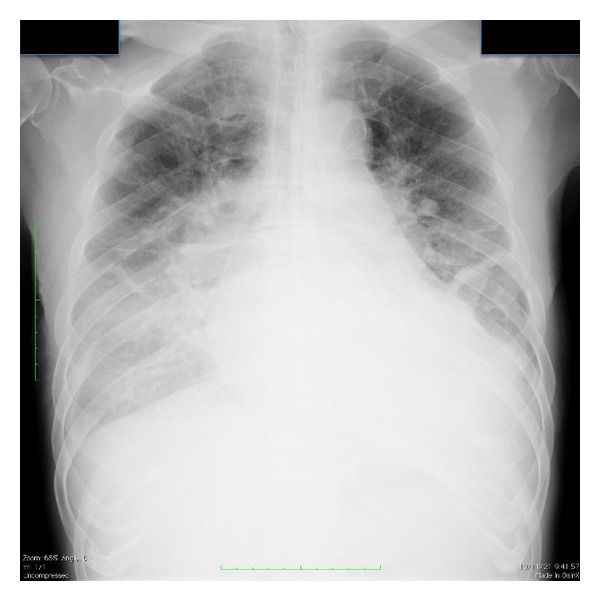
Chest X-ray at admission: significant pulmonary congestion and reduced bilateral lung permeability due to pleural effusion were seen (cardiothoracic rate of 62%).
